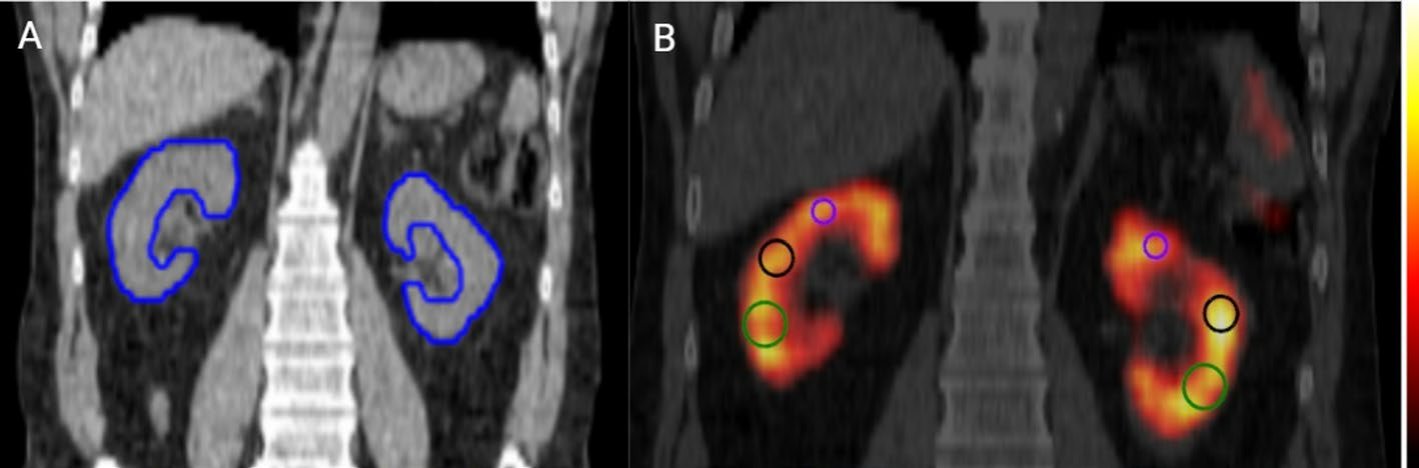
(A) The CT image with manually delineated whole-kidney parenchyma (WKP) volumes (blue). (B) ASCC-SPECT/CT image without post-filter, showing examples of typical locations of SV_4_ (green), SV_2_ (black), and SV_0.6_ (purple) in the kidney parenchyma
